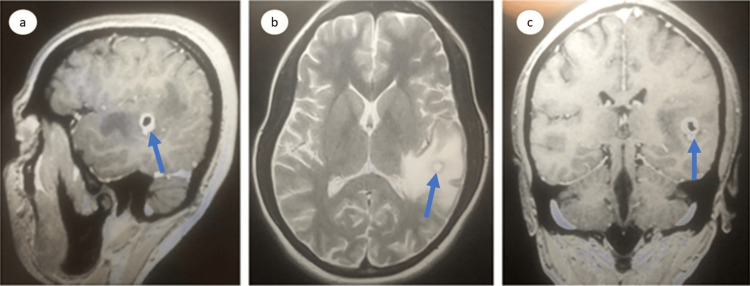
MRI brain with contrast revealing a well-defined rounded discrete lesion seen in the left parietal region as shown in the sagittal (a), trans-axial (b), and coronal (c) sections.
